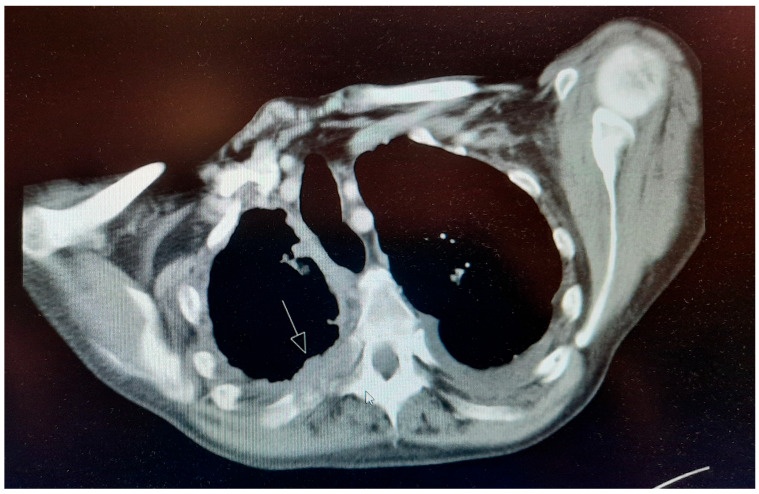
Maximum control of loco-regional and distant disease was achieved in November 2018, i.e., 11 months after initial diagnosis; on CT, only a small pleural effusion (arrow) was visible. The patient has been monitored since then with serial clinical and imaging examinations with CT of the thorax and abdomen, and measurements of tumor marker levels in peripheral blood (CA-15.3, CEA and CA.125), which remained within the normal range; the patient remains progression-free with good quality of life since then, more than eight years after initial diagnosis. This extreme case highlights the necessity of increasing breast cancer awareness among women and awareness of breast cancer symptoms and underscores the impact of popular notions preventing early diagnosis [8]. This study has the inherent limitation of restricted generalizability of conclusions derived from single-case clinical observations. Due to the rarity of ulcerative breast cancer existing evidence is scarce and there are no clear guidelines for treatment available. A recent systematic review on therapeutic strategies for fungating and ulcerating breast cancers [9] showed that published studies have high heterogeneity and low level of evidence. In any case, local care of ulcerative breast cancer lesions is important in order to prevent microbial contaminations [10] and bleeding, as cases of excessive bleeding have been reported, necessitating treatment with local hemostatic agents [11] or radiation therapy [12,13]. In selected cases, sophisticated reconstructive procedures have been reported [14,15]. Previous reports suggest that primary chemotherapy seems to benefit patients with inoperable, ulcerative breast cancer [1,2]. However, individualized treatment should be decided by a multidisciplinary team on a case-by-case basis, based on disease stage, and tumor molecular phenotype according to current clinical guidelines [3,4,5,6].
